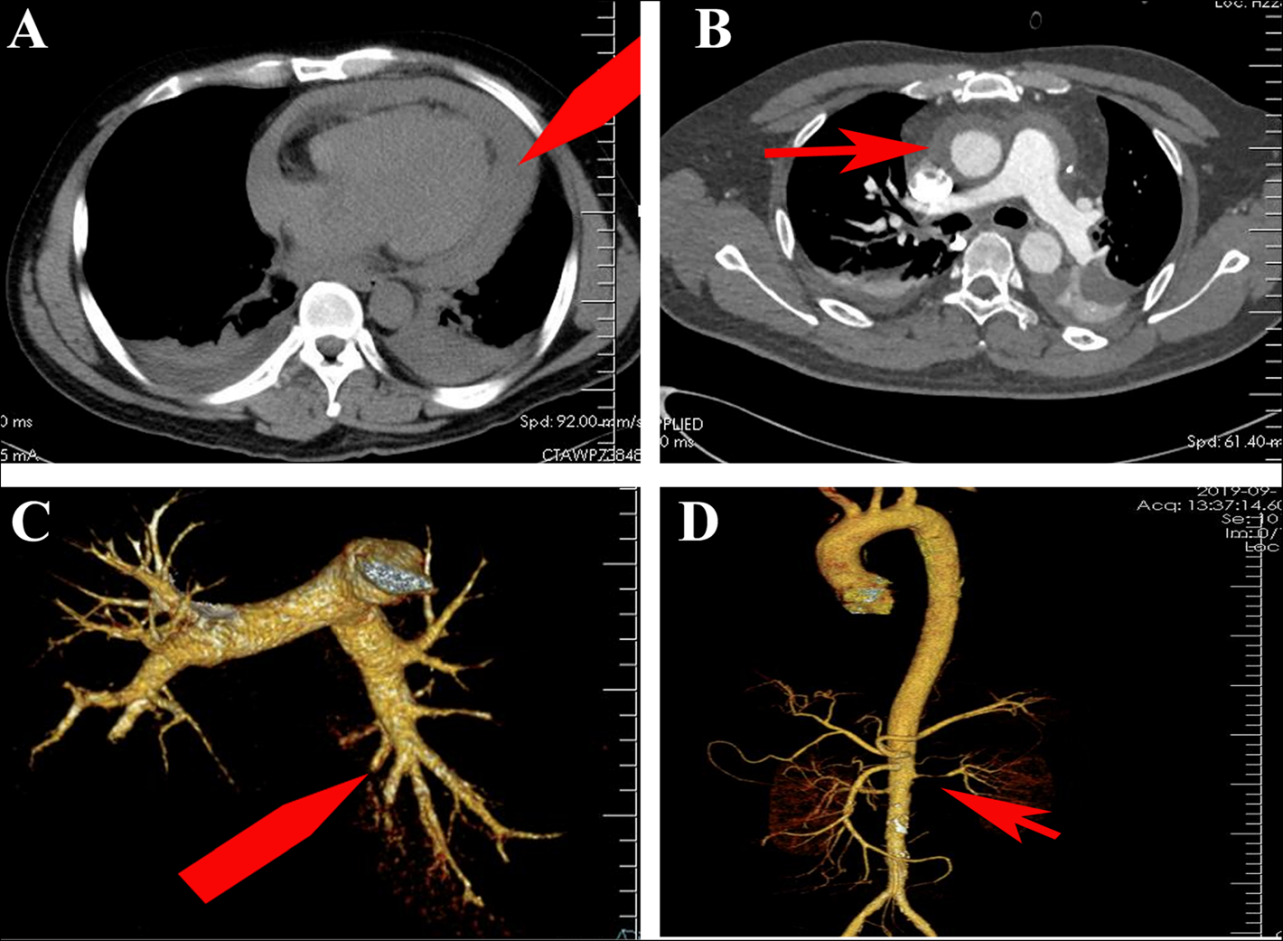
CTA of pulmonary artery, thoracic aorta, and abdominal aorta. a Moderate amount of pericardial effusion (red arrow) and small amount of pleural effusion. b Peripheral hematoma of the ascending aorta (red arrow). c No niche shadow, filling defect, or obvious thrombosis in the bilateral pulmonary arteries (red arrow). d No dissection is detected in the thoracic or abdominal aorta CTA, and stenosis of the LRA is revealed (red arrow)
